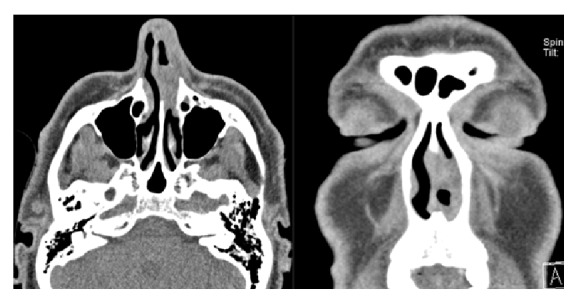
Contrast enhanced CT scan showing a lesion of the inferior turbinate on the left side. Minimal contrast enhancement on the tumor margins.
